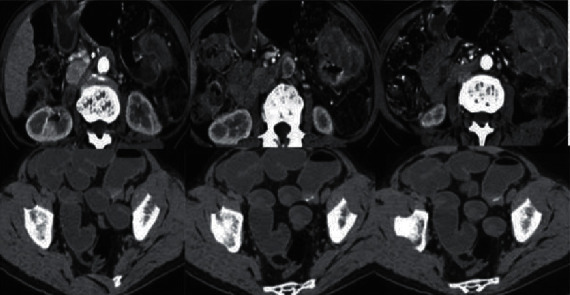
CT images of the patients after being processed by the single-axis super-resolution algorithm.
